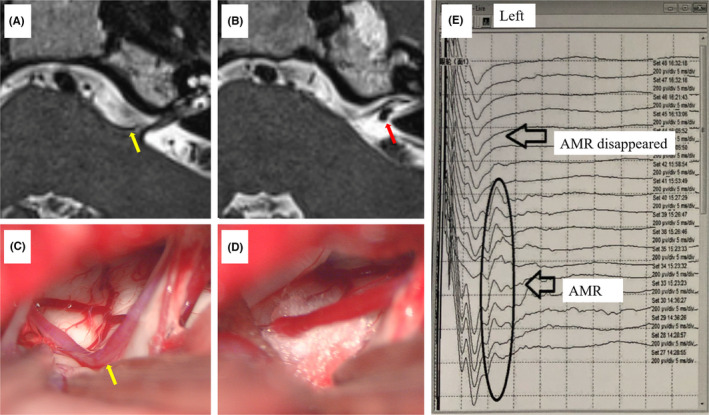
MRI showing the offending vessel at the root entry/exit zone (A) and the small aneurysm of the LA (B). The offending vessel during surgery (C). Teflon felt was placed between involved vessel and facial nerve (D). The AMR disappeared (E). AMR, abnormal muscle reaction; LA, labyrinthine artery; MRI, magnetic resonance imaging
